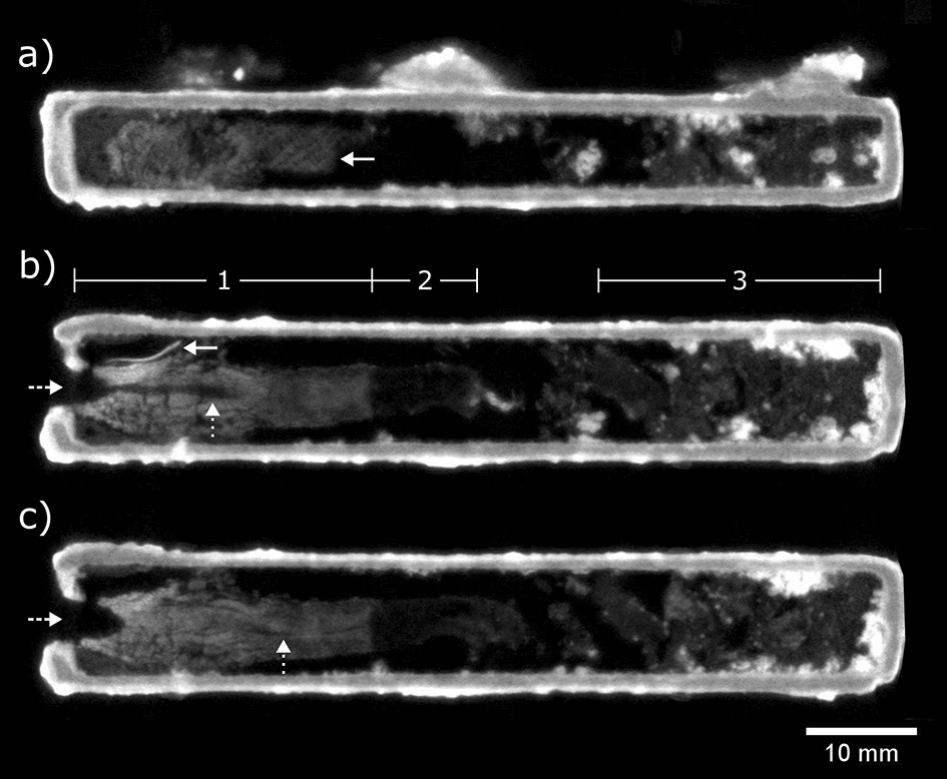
EA49144 neutron CT slices. (a) Side-view, slice 3.2 mm from right side of box (solid arrow: textile in plug). Top-view slices at (b) 4.0 mm and (c) 5.3 mm from base of box, with internal regions (1) plaster plug (2) weakly-attenuating end of plug, (3) fragments. Solid arrow: unidentified object adjacent to plug; dashed arrows: drilled hole in plug; dotted arrows: narrow void extending through plug.
